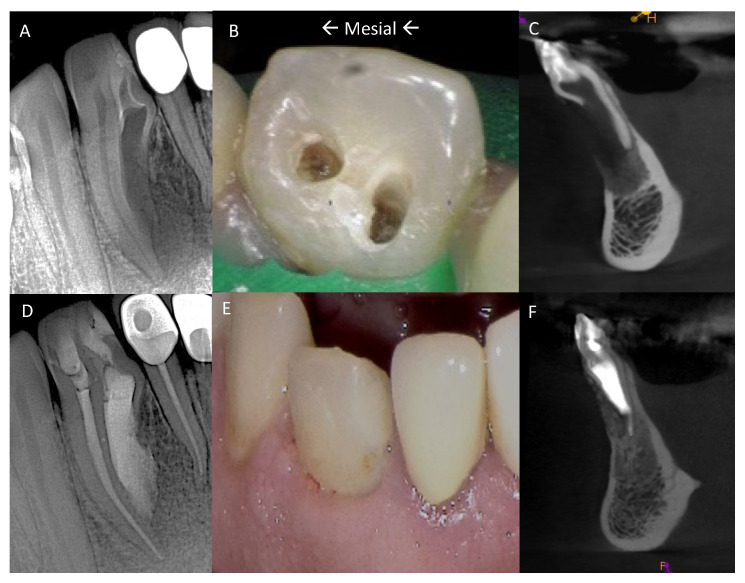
(A) Initial PA taken at endodontic evaluation of tooth #26. (B) Mesial and distal endodontic access cavities. (C) Pretreatment CBCT sagittal section. (D) Final PA after completion of nonsurgical and surgical endodontic treatments and restoration. (E) Photograph of restored tooth #26. (F) Post-treatment CBCT at 6-month follow-up.
